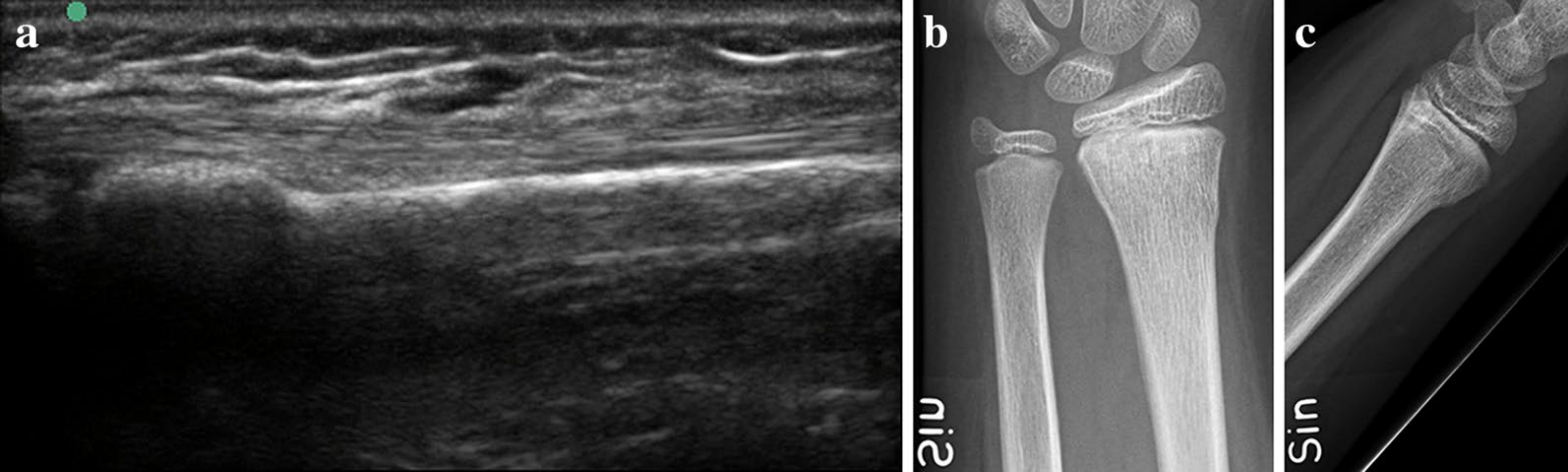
a Infraction/torus fracture of the distal radius, US dorsal longitudinal view. b, c (Same case as a) Infraction/torus fracture of the distal radius on lateral and AP radiographs
